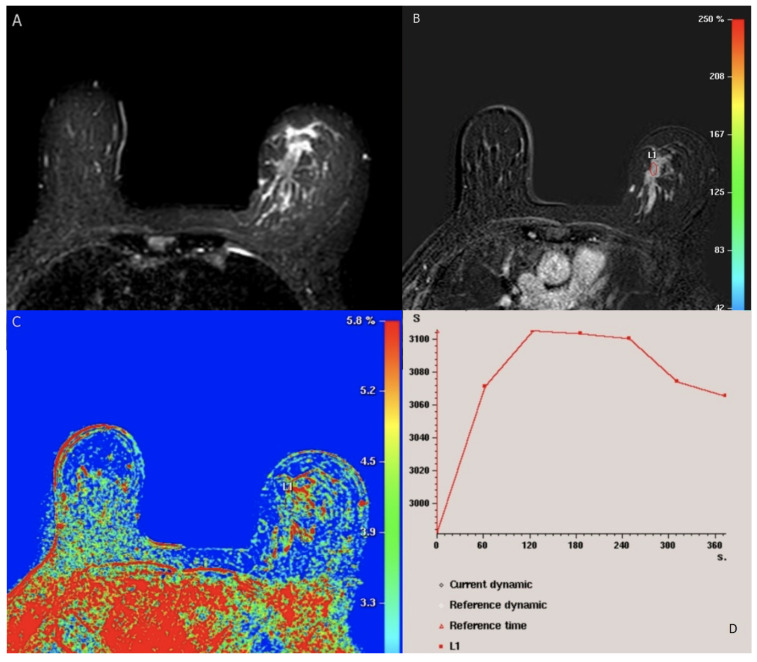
BI-RADS category 5: 49-year-old woman with Luminal (A) (positive ER and PR, negative Her2) lobular carcinoma. Axial fat-saturated T2-weighted MRI shows left breast hyperintense irregular mass. (B) axial T1-weighted fat-saturated dynamic contrast-enhanced MR image shows heterogenous segmental nonmass enhancement of the lesion (L1). (C) kinetic color overlay shows predominant yellow and red colors in the mass indicating plateau kinetics and washout respectively. (D) kinetic signal intensity time curve shows rapid initial phase and washout in the delayed phase. No suspicious axillary LNs.
